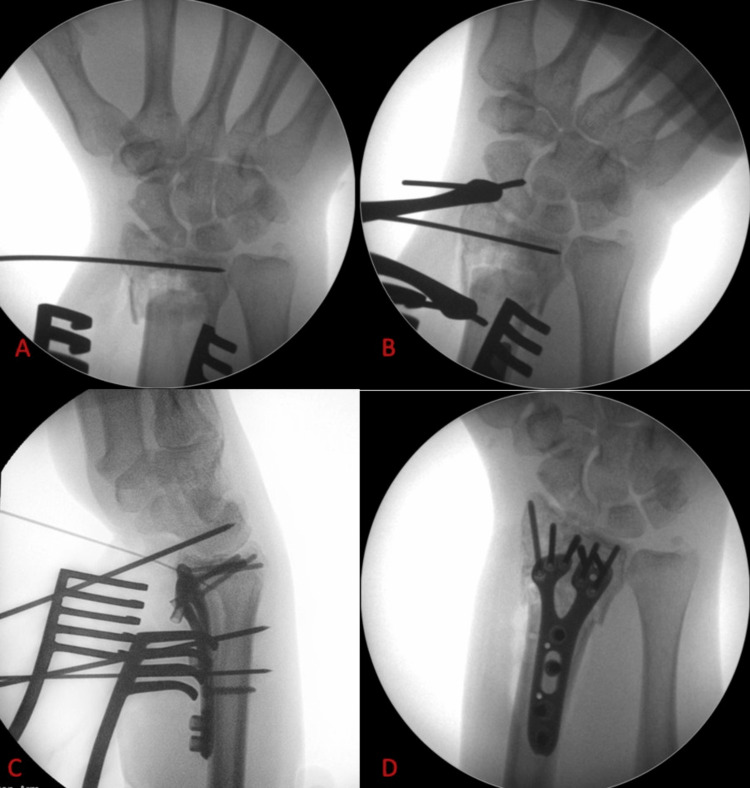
Example of fracture distraction, aiding fracture reduction. (A) Intraoperative radiograph showing loss of height and inclination of comminuted fracture of the distal radius. (B) Intraoperative anteroposterior radiograph showing near-complete correction of the deformity with the application of appropriate ligamentotaxis through a Hintermann distractor, with 1.6 mm K-wires in the radial shaft and the scaphoid. (C) Intraoperative lateral radiograph showing appropriate wire position in scaphoid and radial shaft, and satisfactory reduction of fracture. (D) Intraoperative anteroposterior radiograph demonstrating appropriate reduction of fracture.
